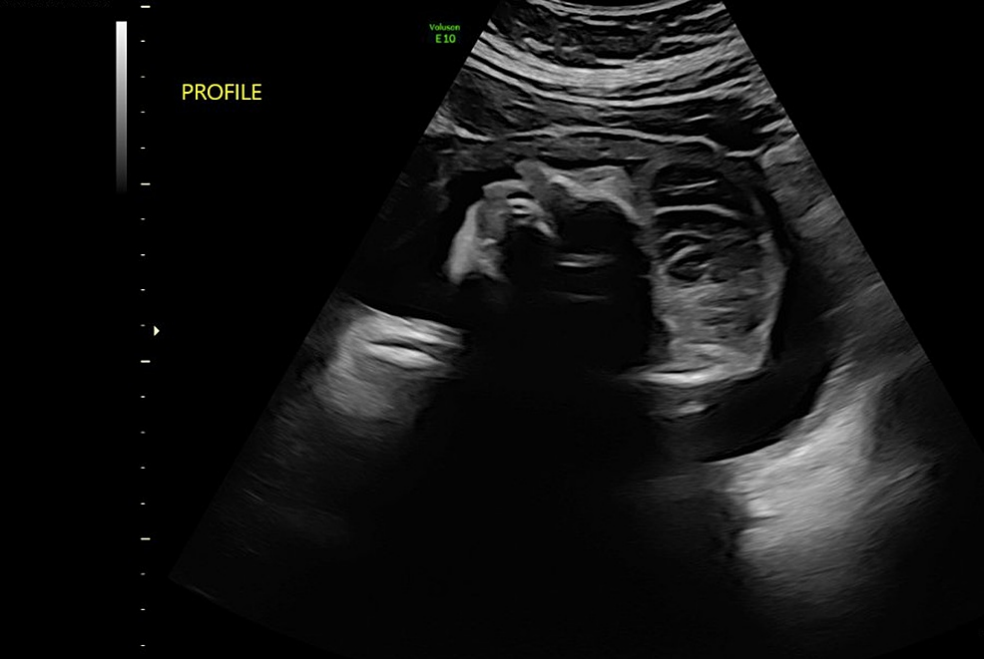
Ultrasound at 30 weeks noting: “acalvaria again noted – the skull bones are absent from the level of the orbit up, there is disorganized brain tissue floating in the amniotic fluid.”
